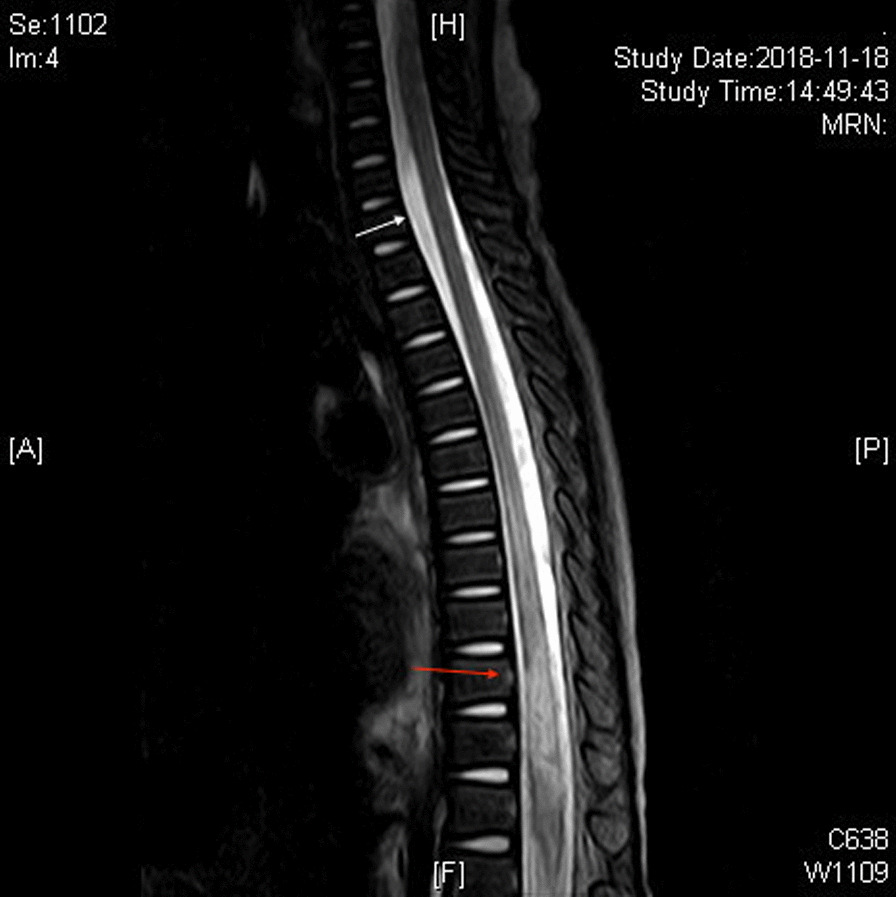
Sagittal T2-weighted magnetic resonance imaging (MRI T2W1) of injured spinal cord. There showed some patchy abnormal shadows with a hyperintense signal of spinal cord below thoracic 1 vertebrae (white arrow), indicating the possibility of spinal cord edema or contusion. There showed also spinal cord swelling at the level of thoracic 10–12 vertebrae (red arrow) with spine fracture, which indicated spinal cord injury (ASIA-A)
